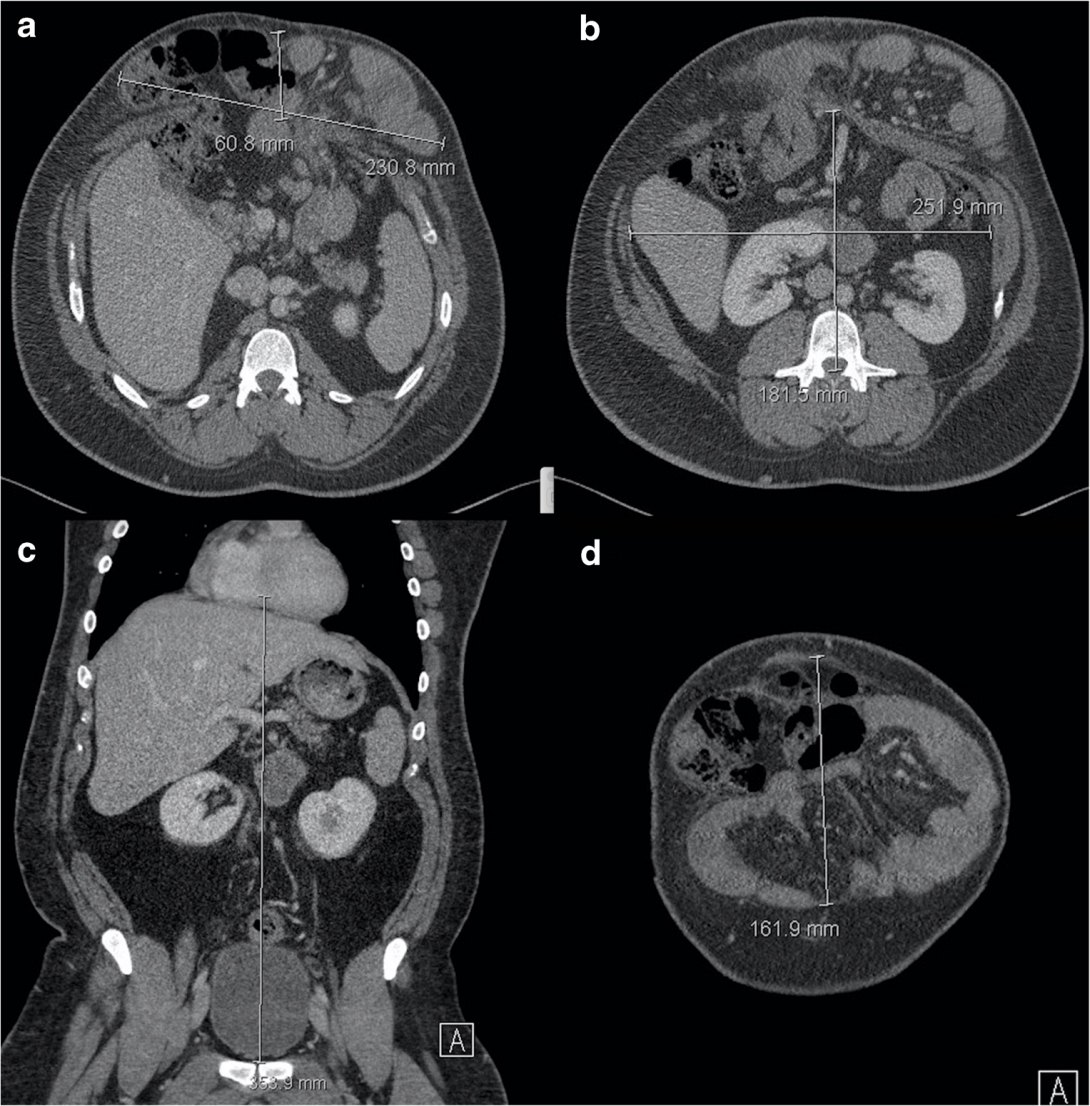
Loss of domain calculation by CT. Figures a and b show measurement of hernia (231 x 61 mm) and abdominal cavity (252 x 182 mm) width and depth respectively. Figures c and d show measurement of abdominopelvic cavity (354 mm) and hernia (162 mm) cranio-caudal length respectively. Estimated hernia sac volume (HSV) = 231 × 61 × 162 × 0.52 = 1,187,026 mm^3^. Abdominal cavity volume (ACV) = 252 × 182 × 354 × 0.52 = 8,442,645 mm^3^. Total peritoneal volume (TPV) is 9,629,671 (i.e. HSV + ACV). Loss of domain by HSV / ACV ratio is therefore = 0.14 and 12% by HSV/TPV, suggesting that hernia repair will not result in serious cardiorespiratory compromise
